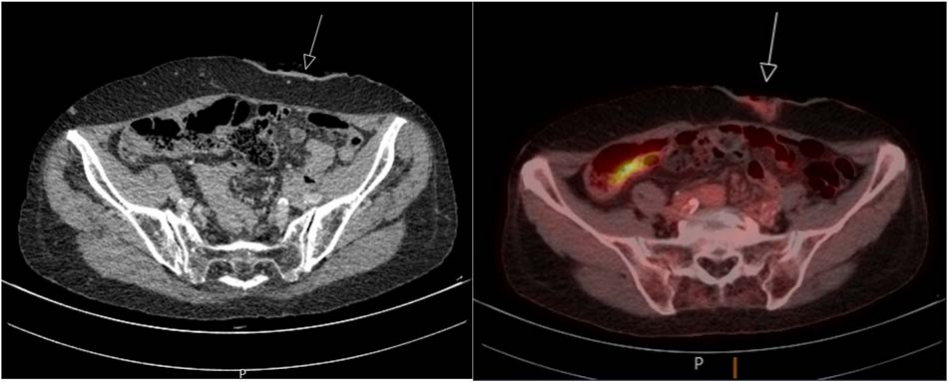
PET/CT scan showing minimal FDG stacking at the colostomy site.
